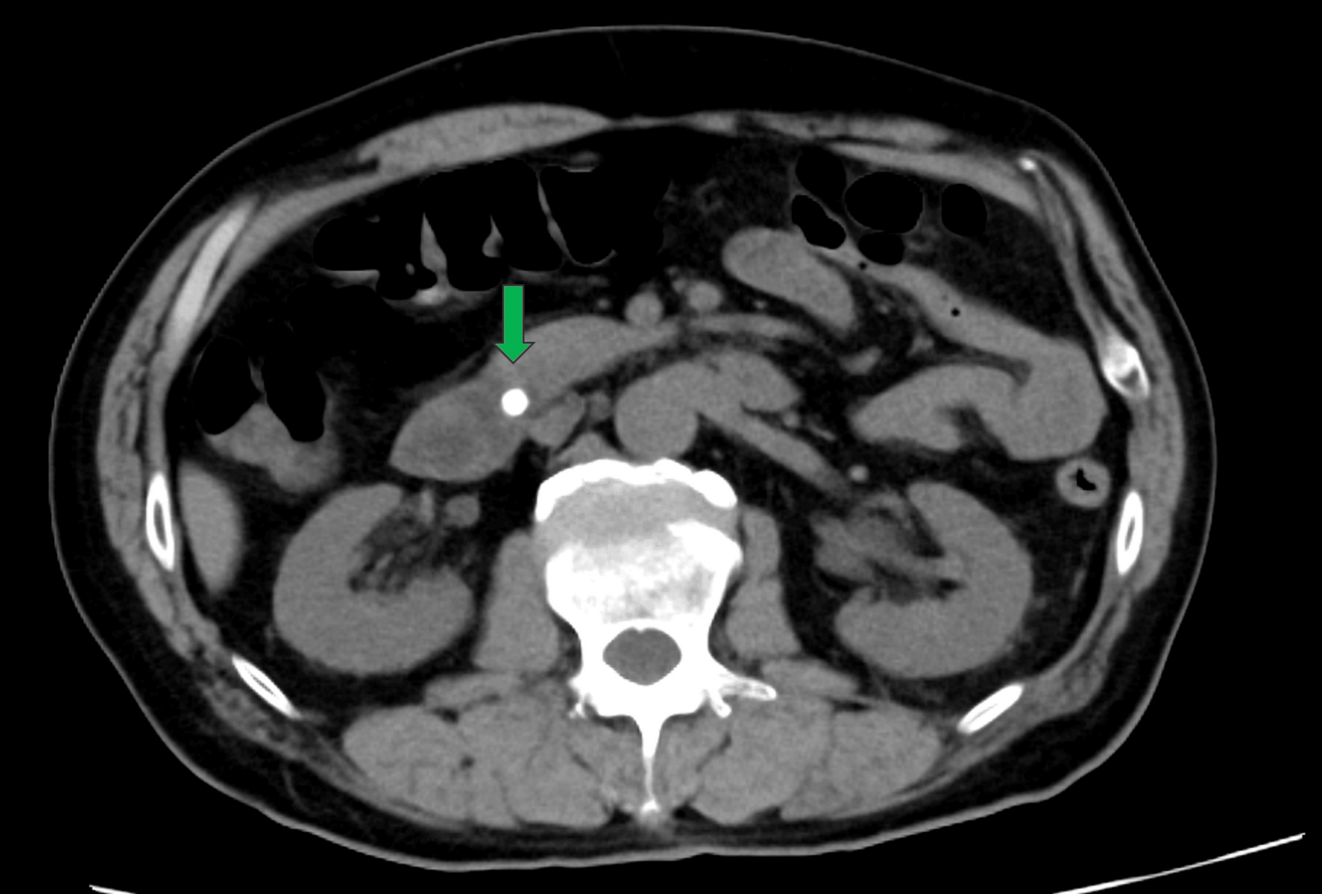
Abdominal CT performed at the referring hospital demonstrating a common bile duct stone (green arrow) without evidence of ascites CT: computed tomography
